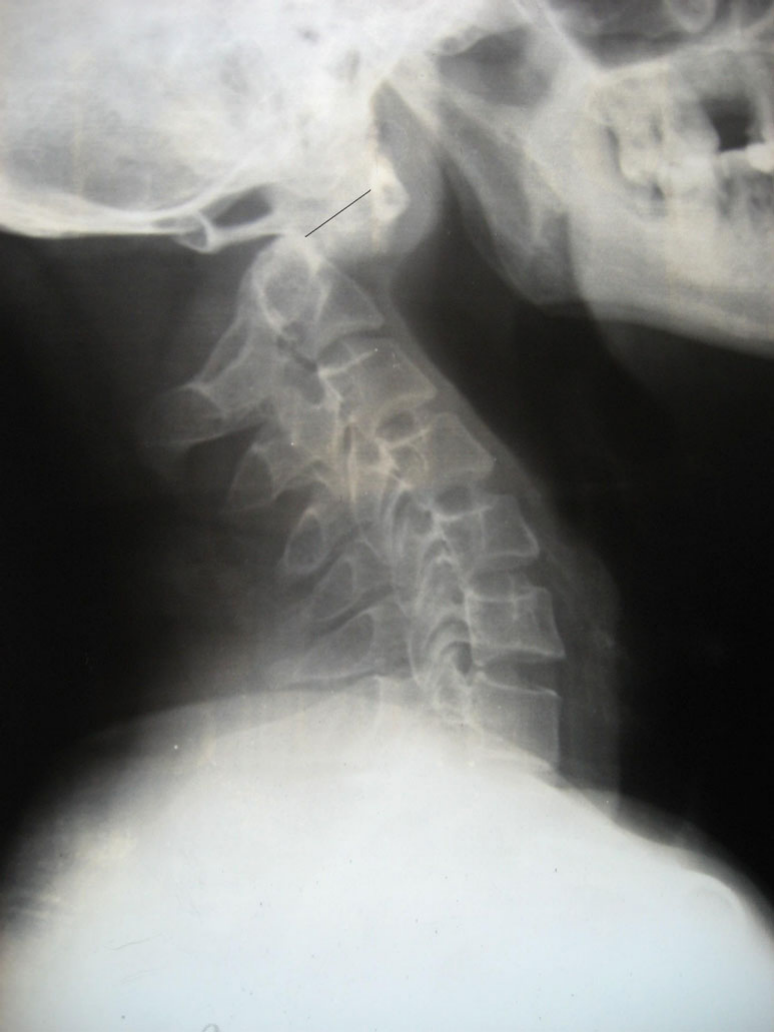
Lateral radiograph of a subject with atlantoaxial instability secondary to odontoid fracture showing marked atlantoaxial displacement.
